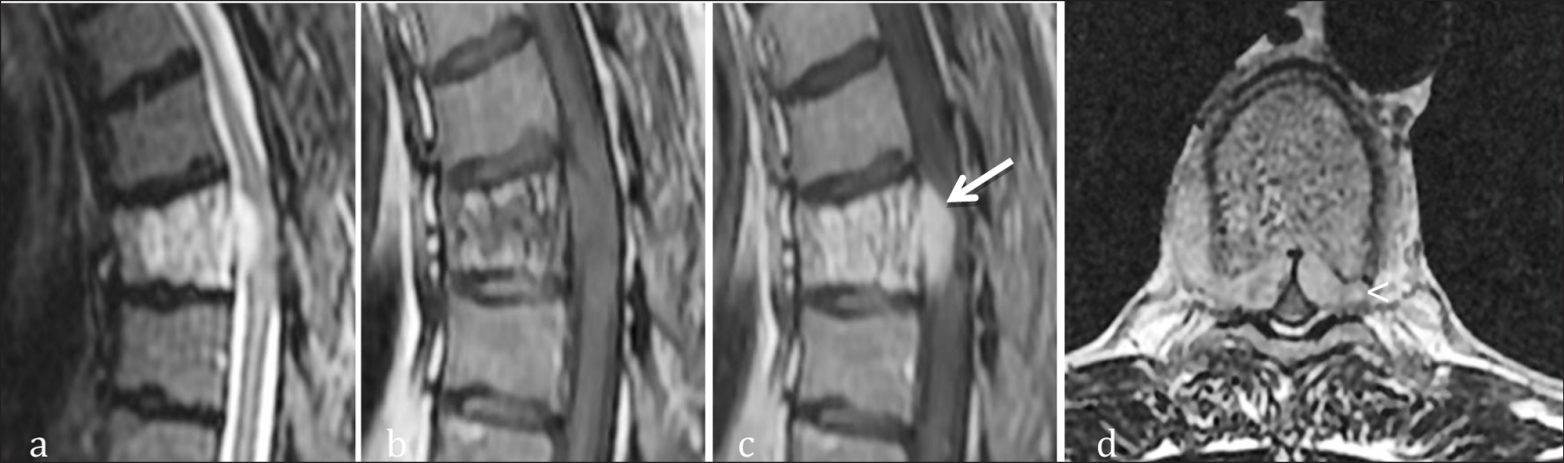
Aggressive hemangioma of T5. Sagittal T2-WI (a), Sagittal T1 WI (b) and sagittal (c) and axial (d) T1-WI after gadolinium contrast administration (c) MR images shows an aggressive hemangioma of T6 with extension into the anterior epidural space (arrow) and soft tissues (arrowhead).
